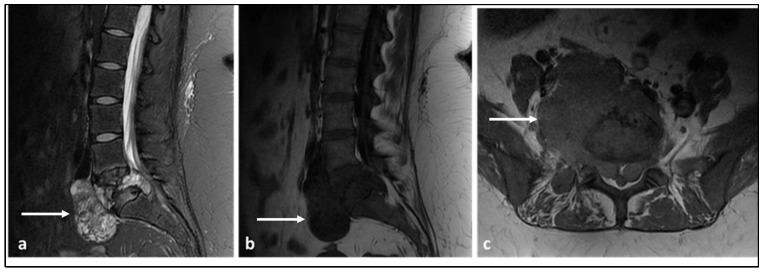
Sagittal STIR (a), sagittal T1 (b) and axial T1 (c) MRI sequences demonstrating a primary vertebral Ewing sarcoma centred in the L5 vertebral body (white arrows). Note the involvement of both pedicles and large extra-osseous soft tissue component extending to the anterolateral paravertebral regions, epidural space and both foramina, the latter causing compression of the thecal sac and exiting L5 nerve roots. The lesion demonstrates the signal characteristics typically seen, with low to intermediate T1 and intermediate to high signal on the fluid-sensitive sequence.
